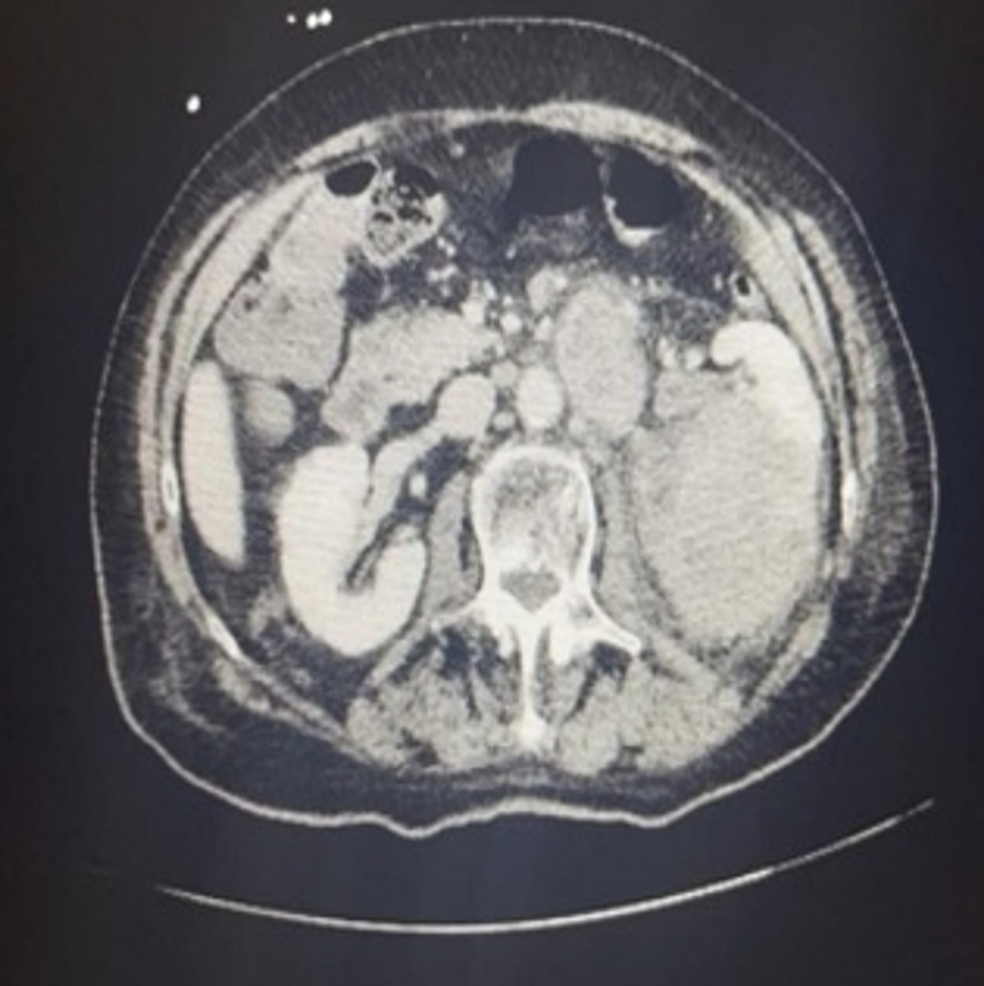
Computed tomography of the large heterogeneous solid renal mass on the left measuring 9.7 x 7.8 x 8.6 cm with extension through Gerota’s fascia and metastases to regional lymph nodes
